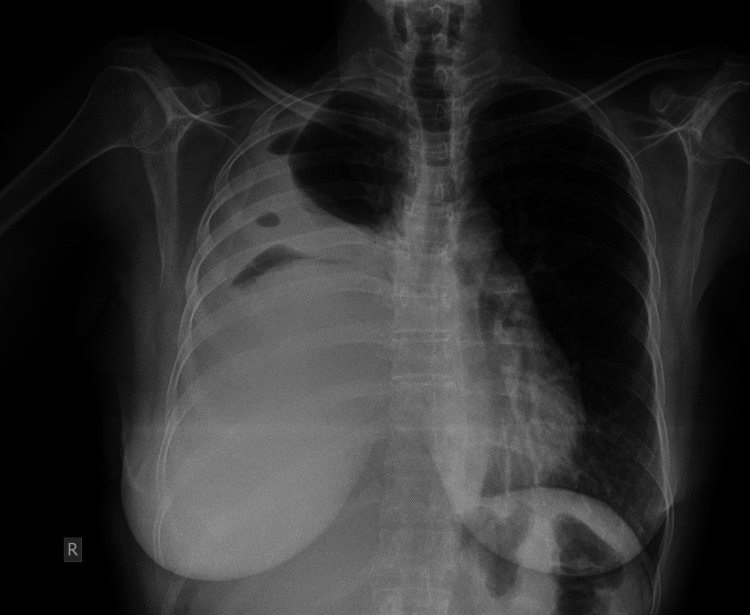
Chest X-ray Anteroposterior chest X-ray showing opacification of 3/4th of the right hemithorax, with a laterally rising border and loculated air pockets within the opacification, suggestive of pleural effusion and loculated pneumothorax
